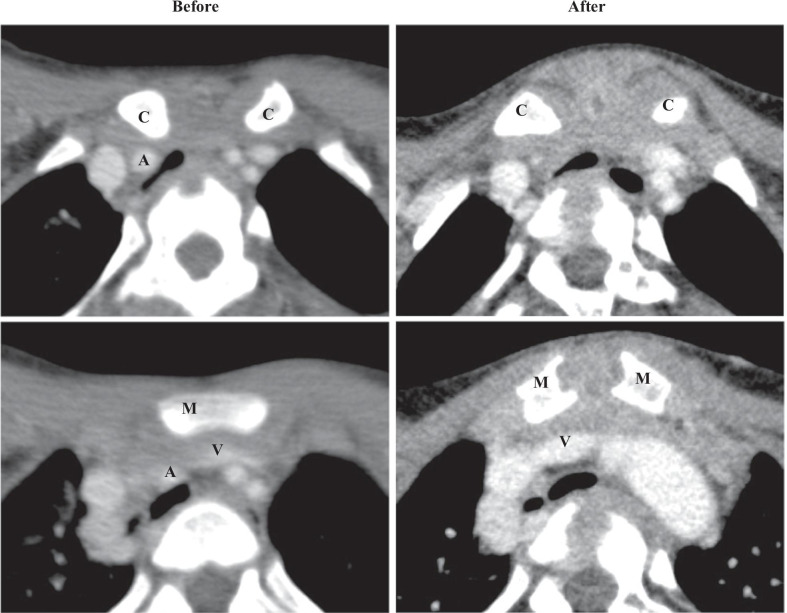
CT imaging before and after transection of the brachiocephalic artery and thoracoplasty in Patient 1.* A* brachiocephalic artery;* C* sternal end of the clavicle;* M* manubrium sterni;* V* brachiocephalic vein
